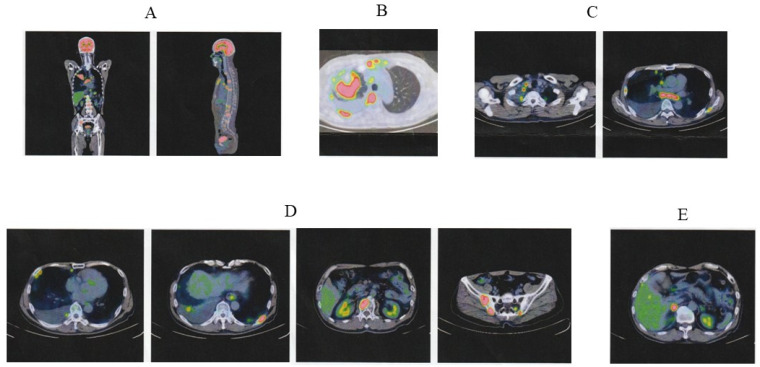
PET-CT images at lung cancer diagnosis. (A) Multiple abnormal signals were observed. (B) A large mass with strong abnormal uptake was prominent in the right upper lobe of the lung. Abnormal uptakes were also detected in pleural effusion and nodules in the right lung and multiple bones (breastbone and vertebral bodies). (C) High metastatic activity was observed in hilar and mediastinal lymph nodes on both sides. (D) Multiple bone metastases were detected in vertebral bodies, bilateral ribs, and bilateral iliac bones. (E) Abnormal uptake was detected in the right adrenal gland. PET, positron emission tomography; CT, computed tomography; ICI, immune checkpoint inhibitor.
